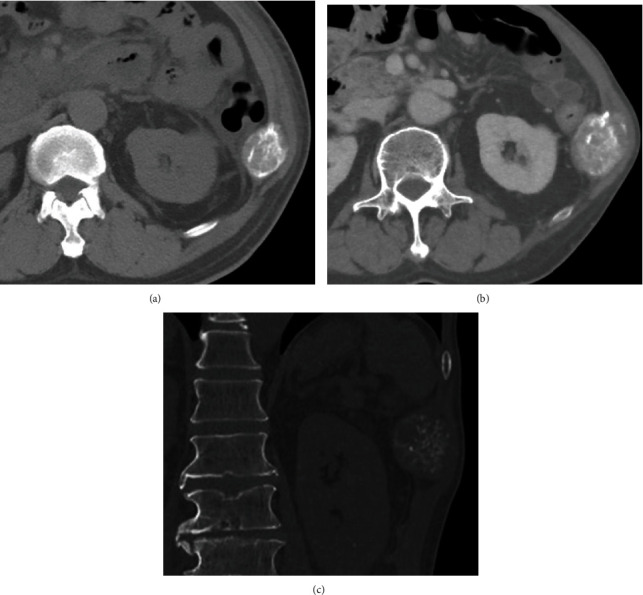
(a) Computed tomography (CT) image of the tumor showing cortical destruction and calcification in the 11th rib. (b) The tumor slowly grew at three-year intervals. (c) Calcification resembled the ring and arc calcifications observed in chondrosarcoma on CT.
